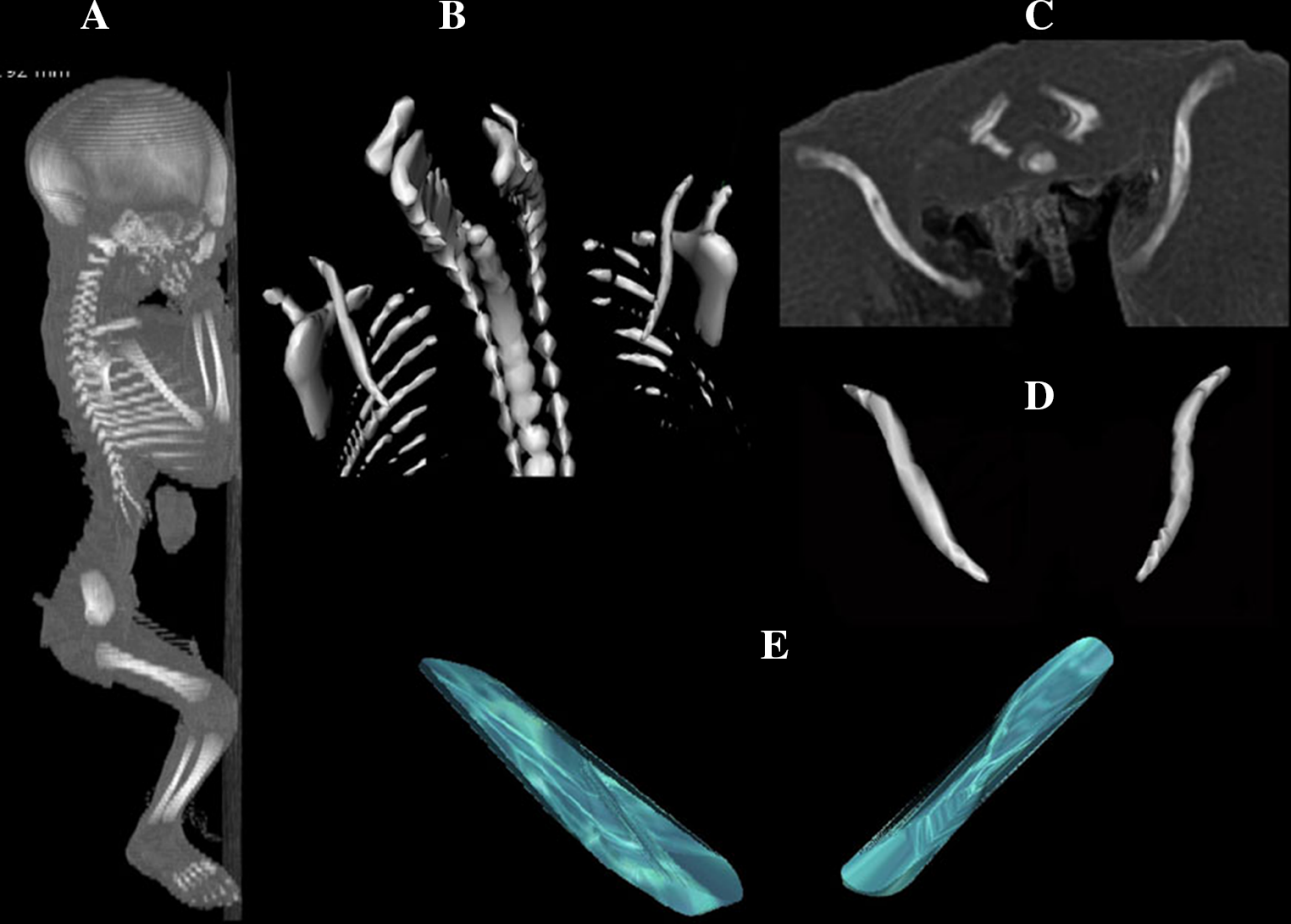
CT of a male fetus aged 26 weeks (in the sagittal projection) recorded in DICOM formats (*a*) with further reconstructions of its clavicles in superior-anterior (*b*) and horizontal (*c*, *d*) projections, including its fused primary ossification centers (*e*), assessed by Osirix 3.9
